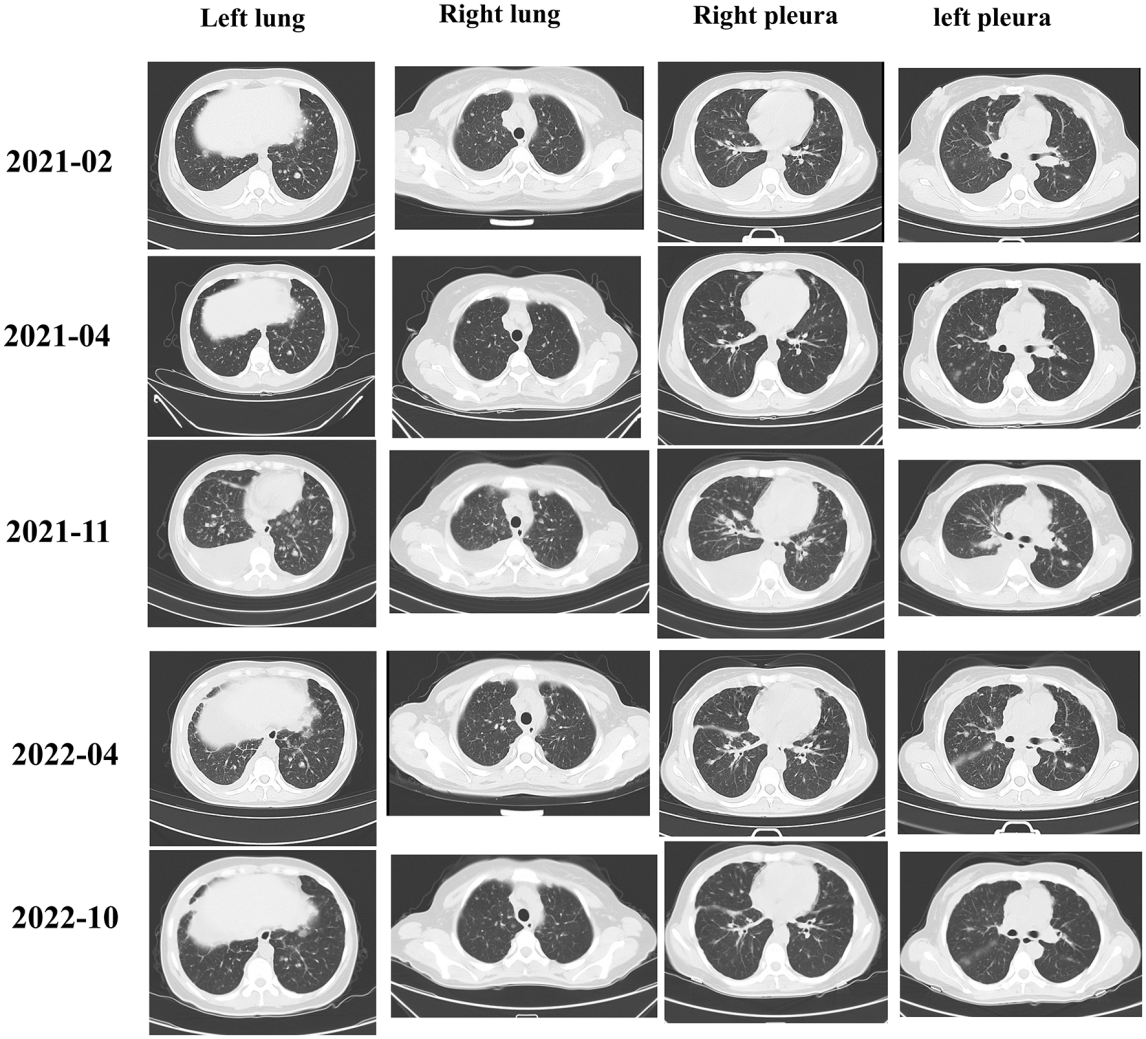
The patient had new metastatic lesions after surgery but showed a response during immunotherapy. At 3 months after surgery, CT revealed metastatic lesions in both lungs and the pleura. The lung lesions were stable until November 2021 after sintilimab and anlotinib treatment. With the switch to pembrolizumab and axitinib, the lung lesion showed a partial response in October 2022
